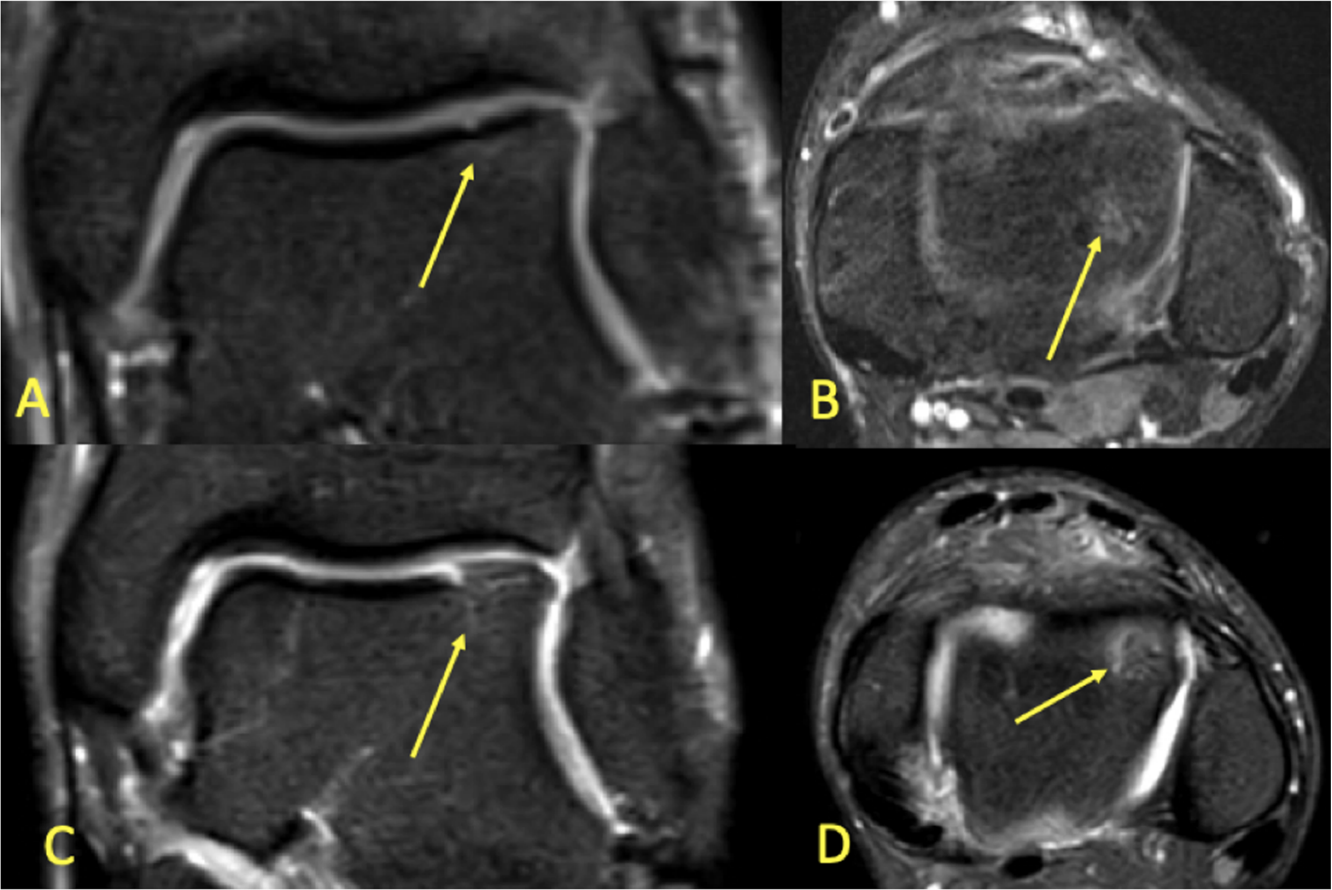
A 19-year-old handball player with 3-month history of ankle pain imaged for suspected anterior tibiofibular ligament rupture and stress fracture. a, b On the initial MRI, the cartilage lesion (arrow) was missed, and neither an anterior tibiofibular ligament rupture nor a stress fracture was detected. c, d Because of persistent pain preventing training, MRI was repeated after 8 months. A chondral lesion with subchondral BME was visible and could be identified retrospectively on the previous MRI (arrows)
